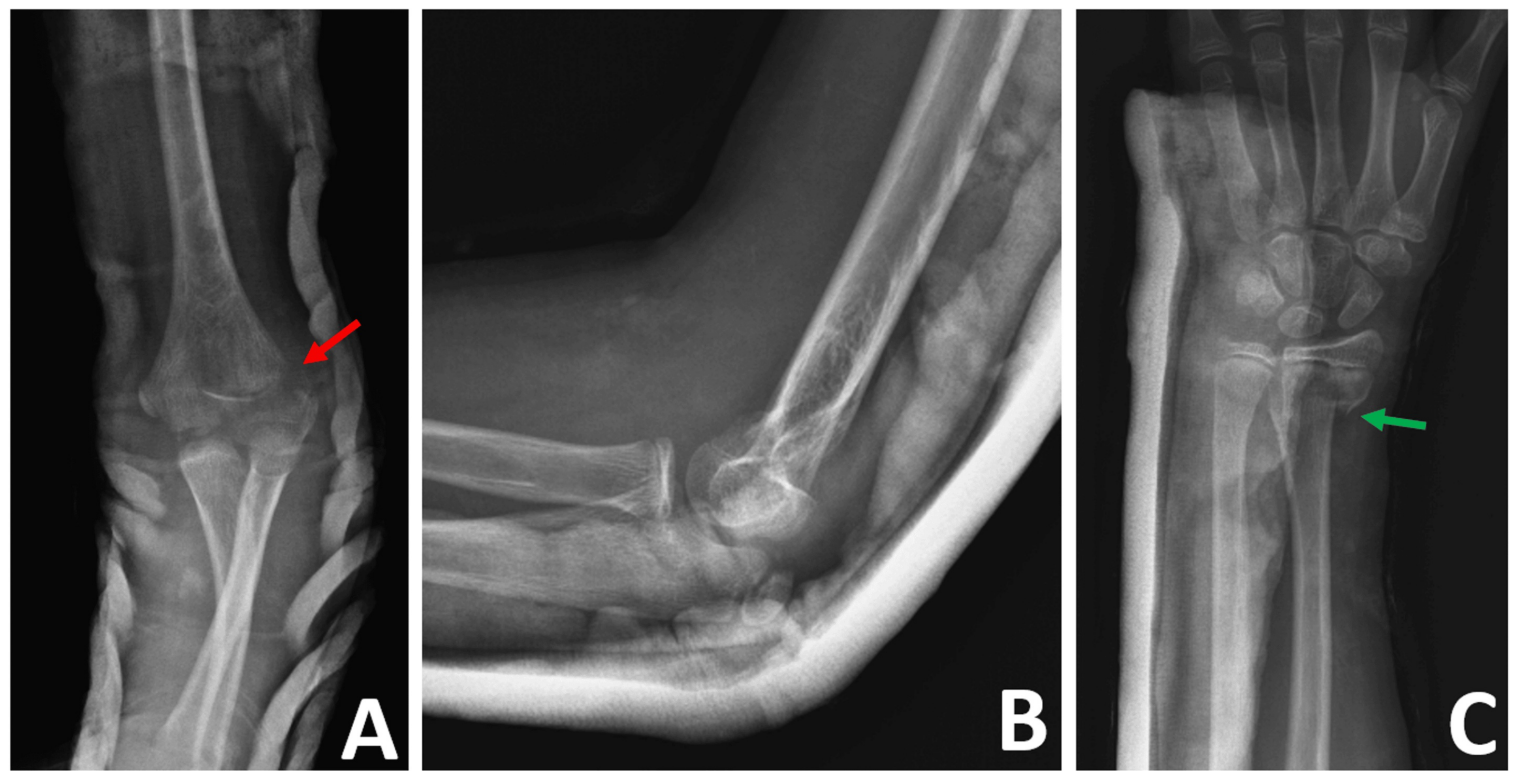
Preoperative radiographs of the left elbow and wrist after closed reduction and splinting (A) Anteroposterior radiograph of the left elbow shows a Milch type II fracture of the lateral condyle, with displacement greater than 4 mm. Red arrow indicates the fracture line. (B) The lateral radiograph demonstrates that the elbow is in a reduced position after a closed reduction maneuver. (C) Anteroposterior radiograph of the left wrist shows a distal radius metaphyseal fracture (green arrow).
